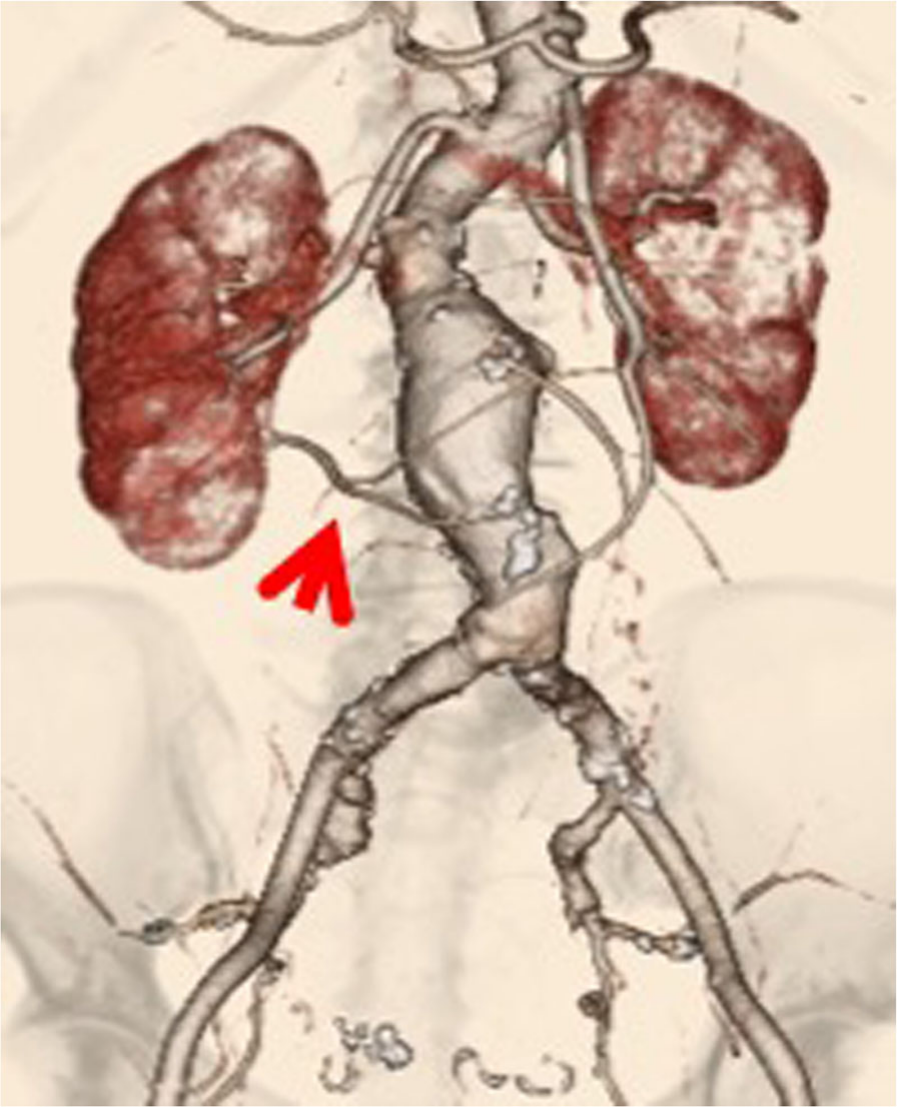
Preoperative enhanced CT. The maximum diameter of the AAA was 53 × 57 mm, and the diameter of the right ARA was 4 mm (*arrow*)
